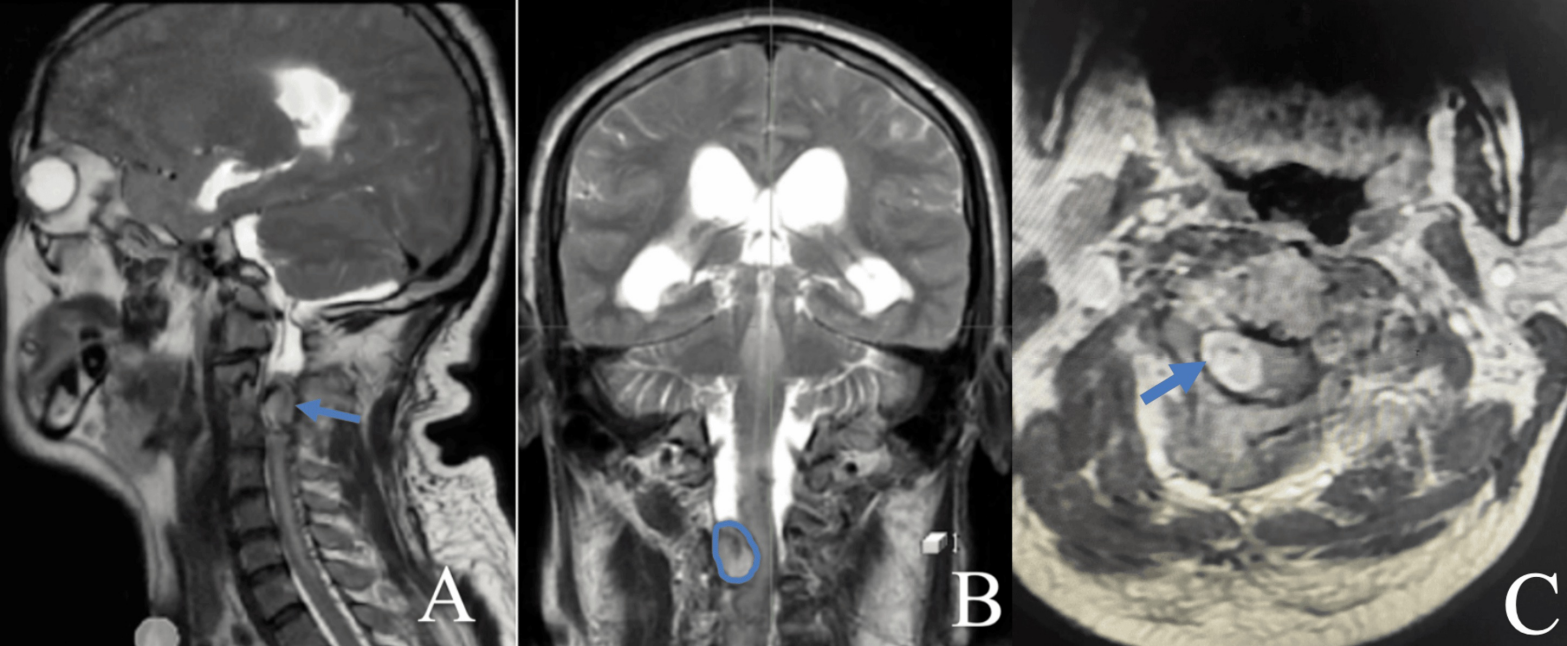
Cervical MRI: T2-weighted images, sagittal (A), coronal (B) and axial (C) planes. Right-sided cervical extramedullary lesion at the C3-C4 level (arrow, highlighted in blue) appears heterogeneous with mixed signal on T2 (hypersignal associated with a central area of hypointensity consistent with hemosiderin deposition). This lesion is exerting a mass effect on the cervical spinal cord that appears displaced to the left.
